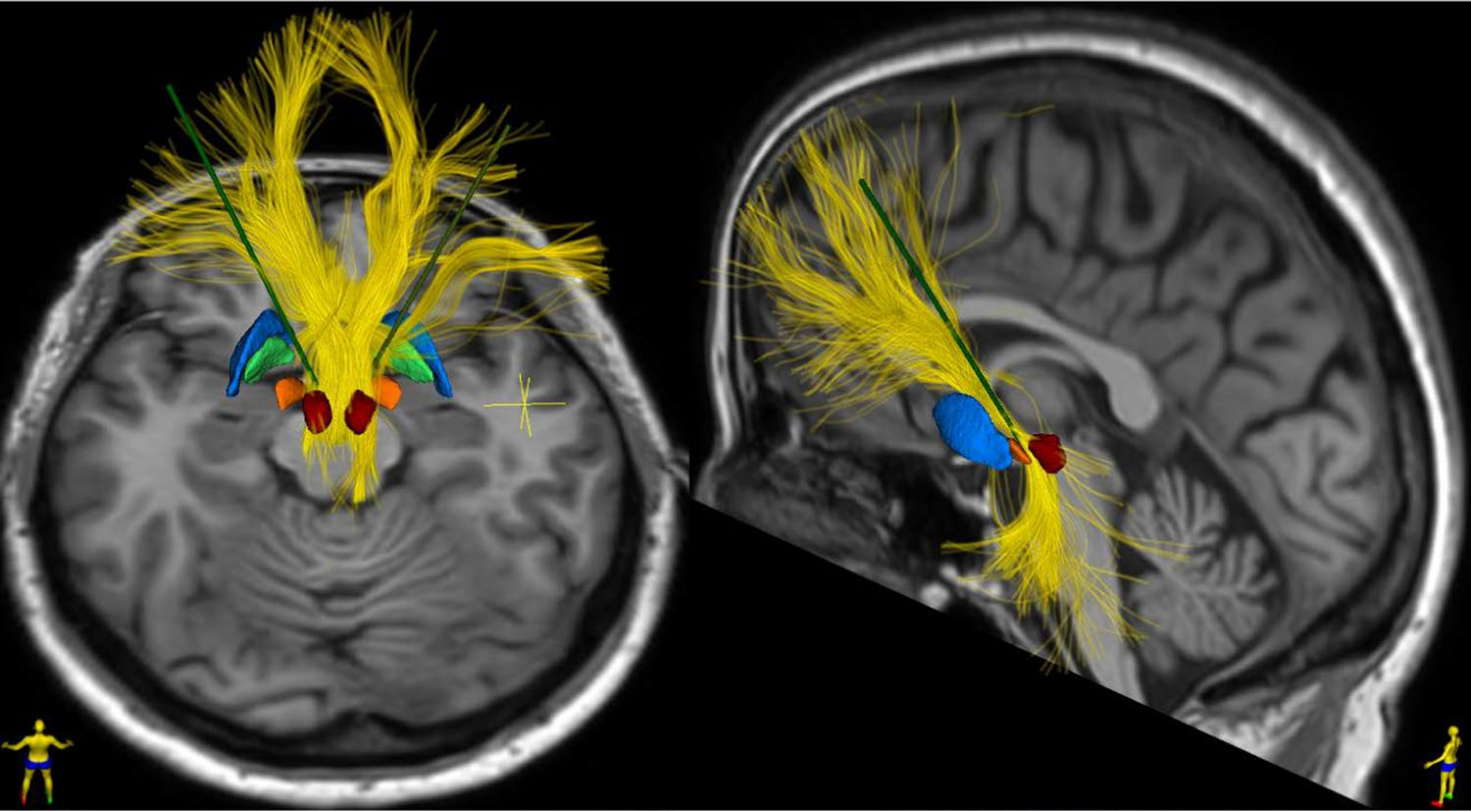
Axial and sagittal view of a T1W brain MRI from a 58-year female with TRD with bilateral electrodes (green) placed in the medial forebrain bundle (MFB, yellow). UKF two-tensor tractography model [127] is used to isolate the MFB and electrodes are stereotactically placed directly within the white matter bundle. The MFB is located medial to the subthalamic nucleus (orange) and anterior to the red nucleus (Red). Significant anti-depressant effects were seen post-DBS at 1 year with reduction in MADRS scores by 66% from preoperative baseline
